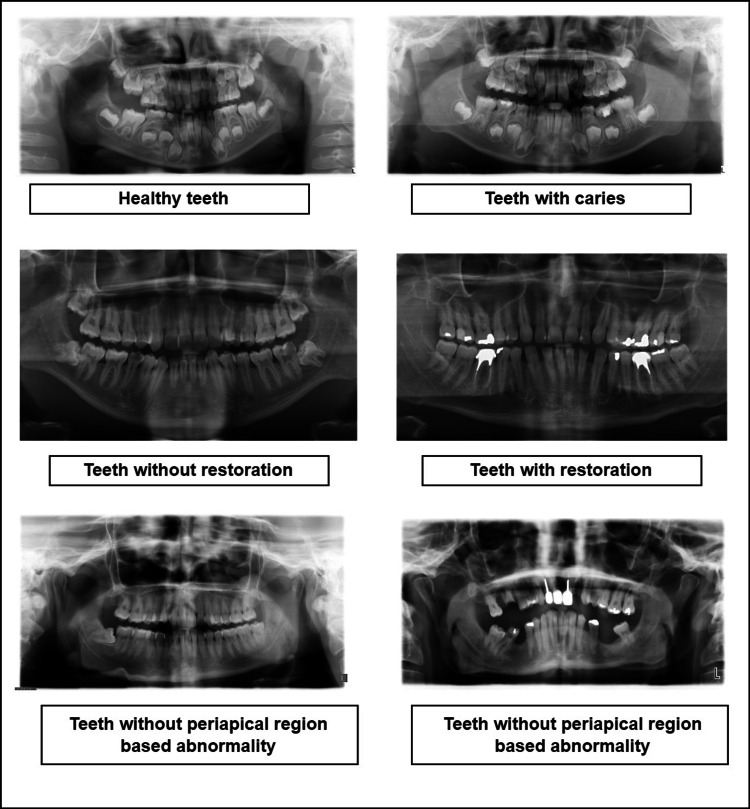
Sample dental radiographs used in the experimental analysis of the study
